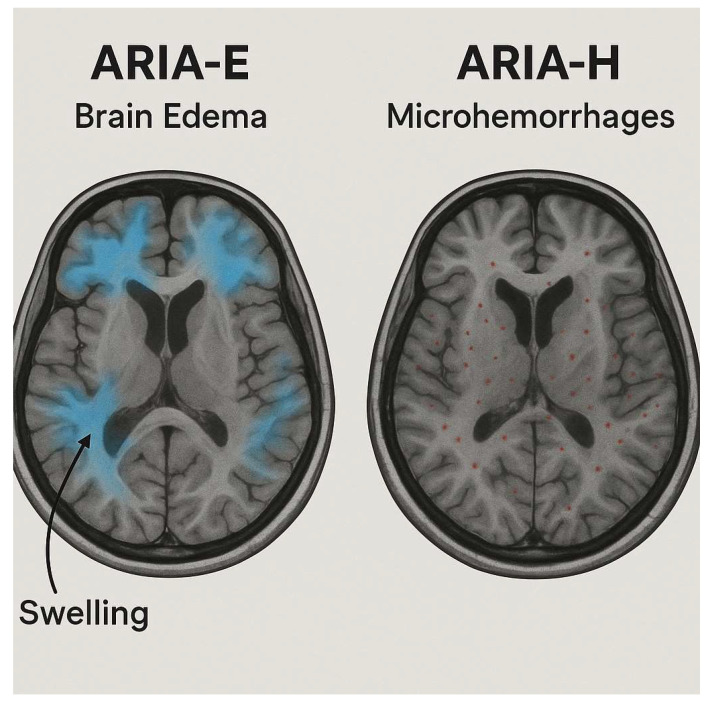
Representative MRI scans showing ARIA. On the left, ARIA-E indicates brain edema characterized by areas of swelling (highlighted in blue). On the right, ARIA-H displays microhemorrhages marked by small red dots throughout the brain. Created in BioRender. Christodoulou, R. (2025) https://BioRender.com/801nbt2 (accessed on 2 September 2025).
